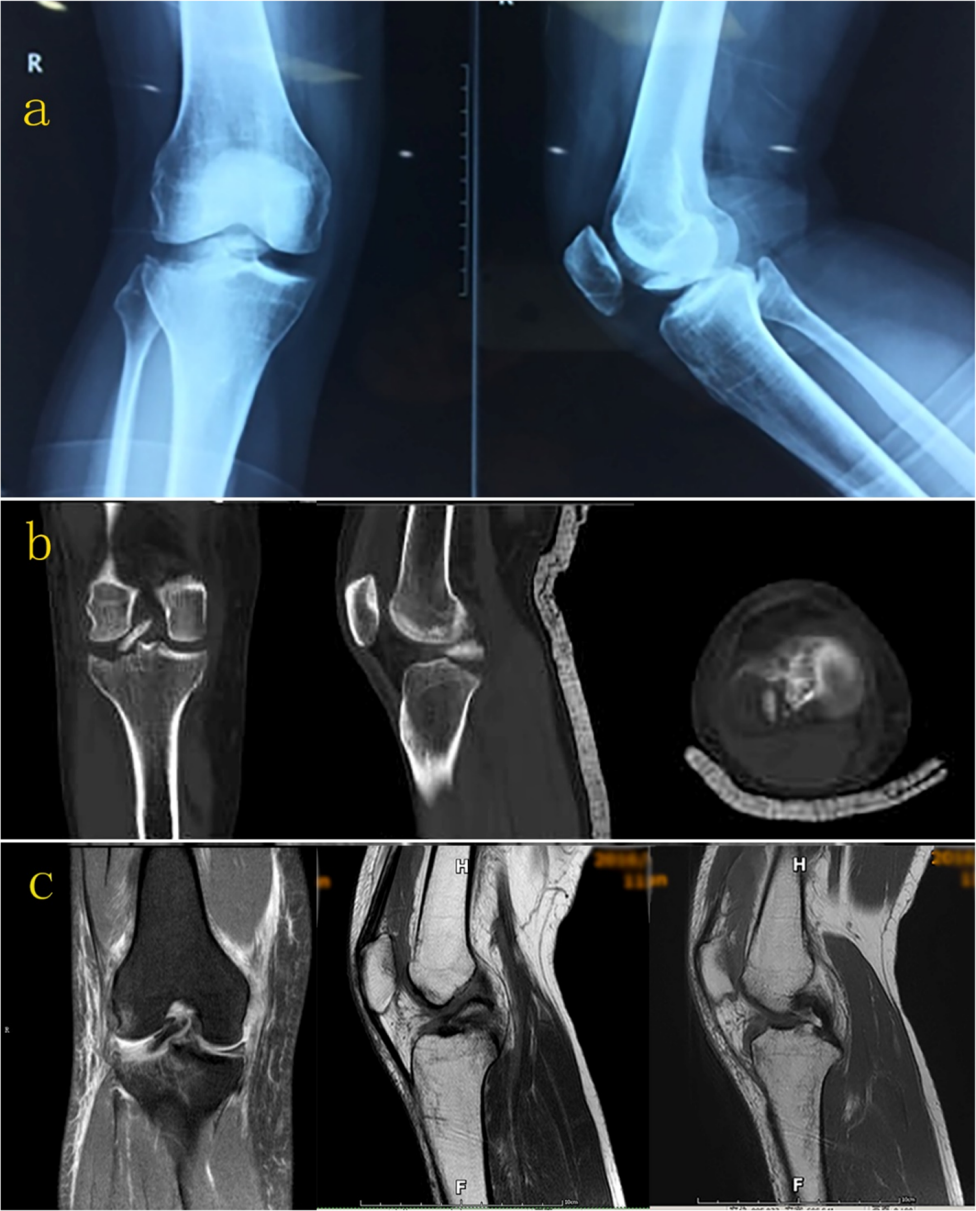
(a) On anteroposterior and sagittal X-rays, irregularities were observed in the lateral tibial plateau, and a high-density shadow was seen in the femoral intercondylar fossa. (b) CT imaging demonstrated that the lateral tibial plateau fracture was involved in a posterolateral location only, and the fragment was inserted into the femoral intercondylar fossa. (c) MRI showed that the ACL and PCL were intact, while the lateral meniscus and MCL exhibited injurious signal intensity
